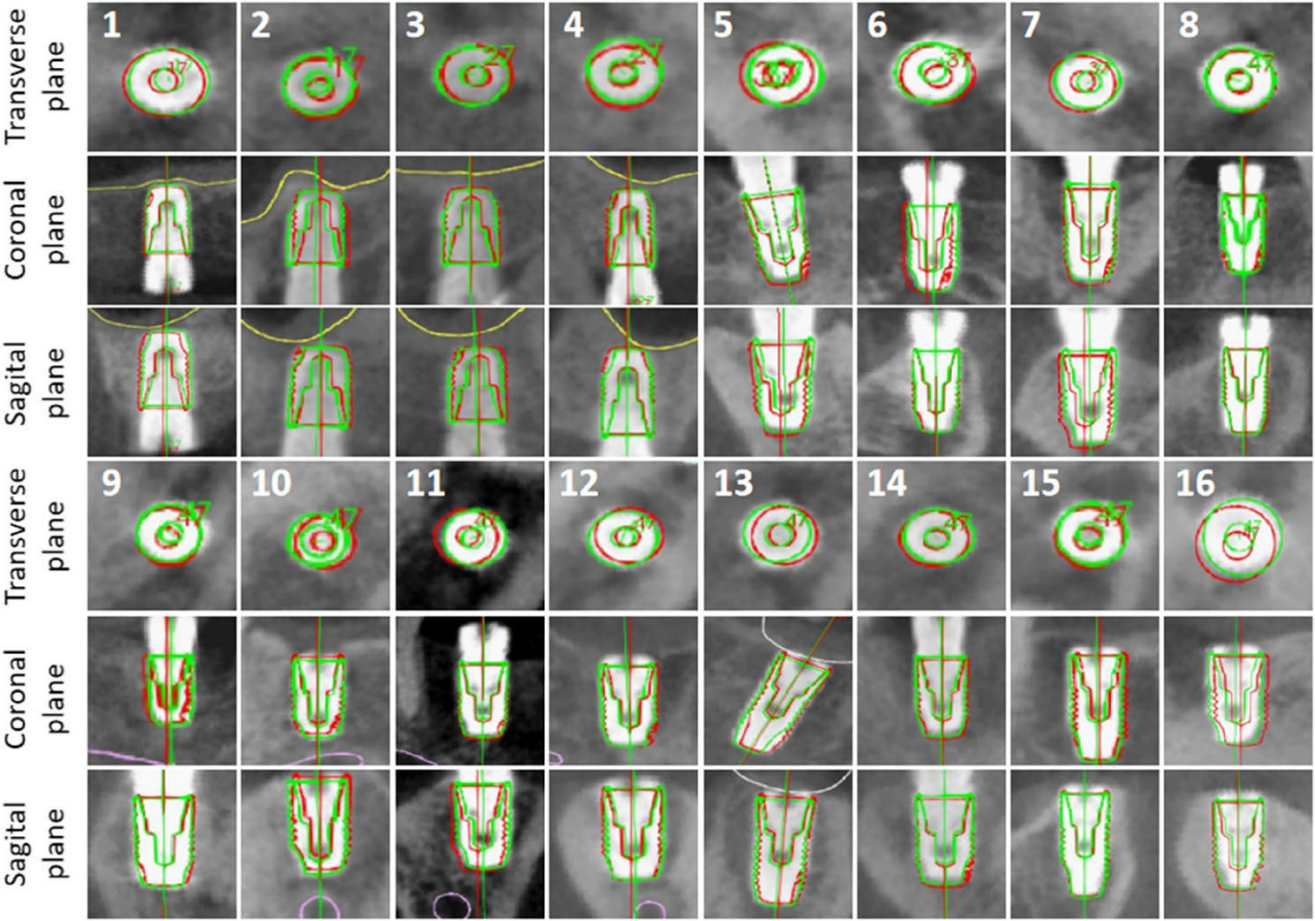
CBCT fusion images of the planned and placed implants for each patient. The planned implants are outlined in red, and the placed ones are outlined in green
